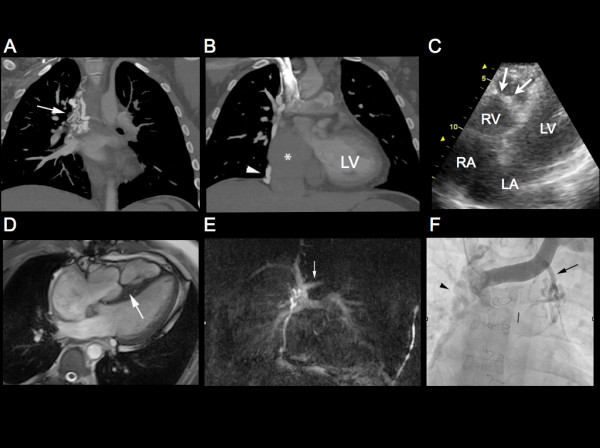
(A) Maximum intensity projection pulmonary arterial phase CT images of the thorax reveal extensive venous collaterals arising from the superior vena cava (arrow), (B) which join to the inferior vena cava (arrowhead) and markedly dilated IVC and right atrium (star). (C) An apical 4 chamber view on transthoracic echocardiography, demonstrating an echodense muscular band within the RV, consistent with a DCRV. (D) Balanced steady state free precession (B-SSFP) cine true axial reveal a thickened muscular band extending across the right ventricle (large arrow). (E) Subtracted MR pulmonary angiogram via right antecubital vein injection reveals a patent Glenn shunt and tortuous venous collaterals extending from the SVC to IVC as demonstrated on the CT examination. Note there is only very minimal reflux of contrast into the proximal left innominate vein (arrow). (F) Selective venogram of the left innominate vein demonstrates the large venous collateral draining into the left superior pulmonary vein (arrow) along with the previously demonstrated SVC-IVC venous collaterals (arrowhead).
